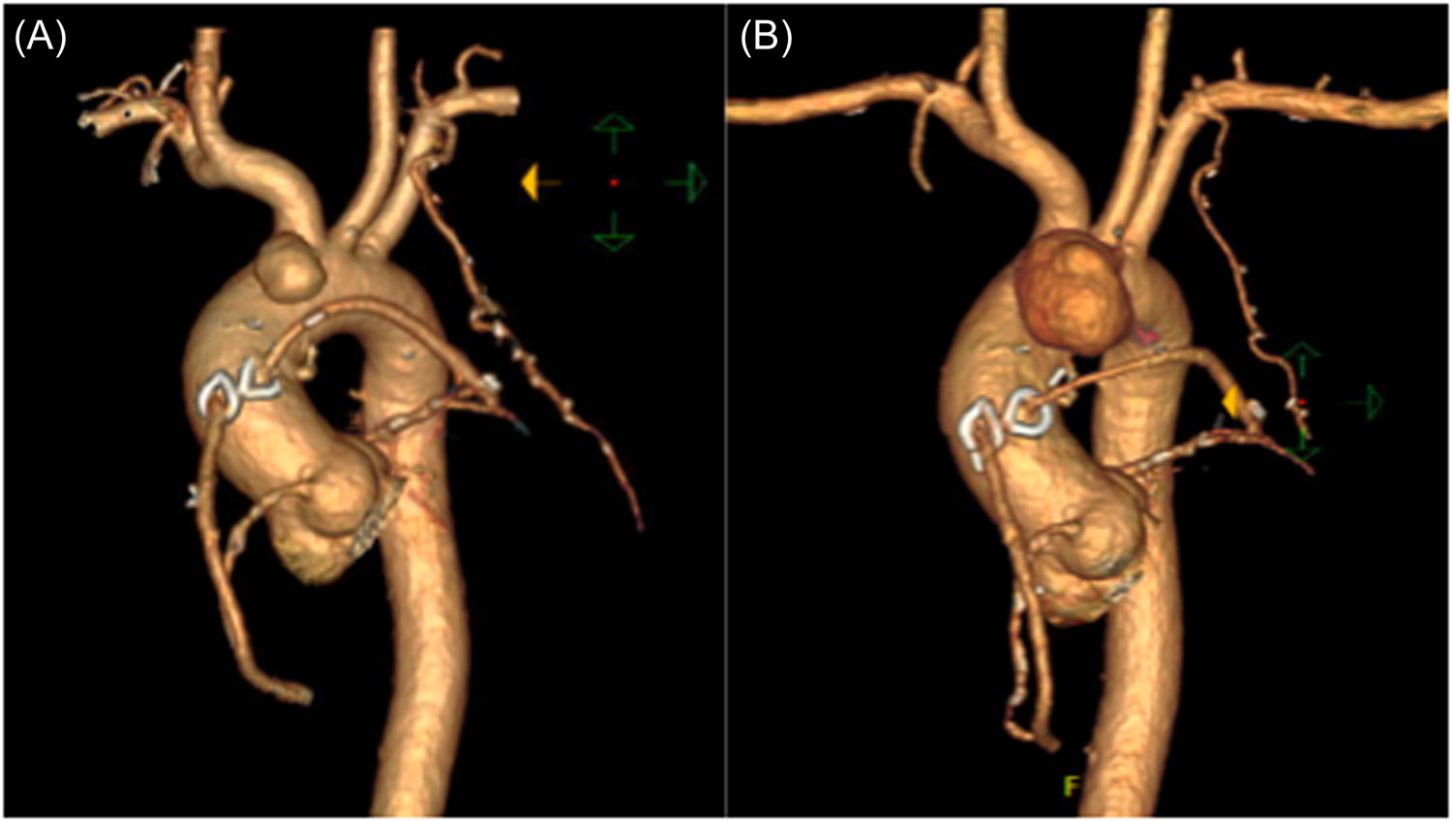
(A) 3D CT reconstruction demonstrating the aortic pseudoaneurysm when initially diagnosed in communication with the former aortic cannulation site. (B) A further 3D CT reconstruction demonstrates enlargement of the pseudoaneurysm observed 12 days later with interval reimaging. CT, computed tomography; 3D, three dimensional.
